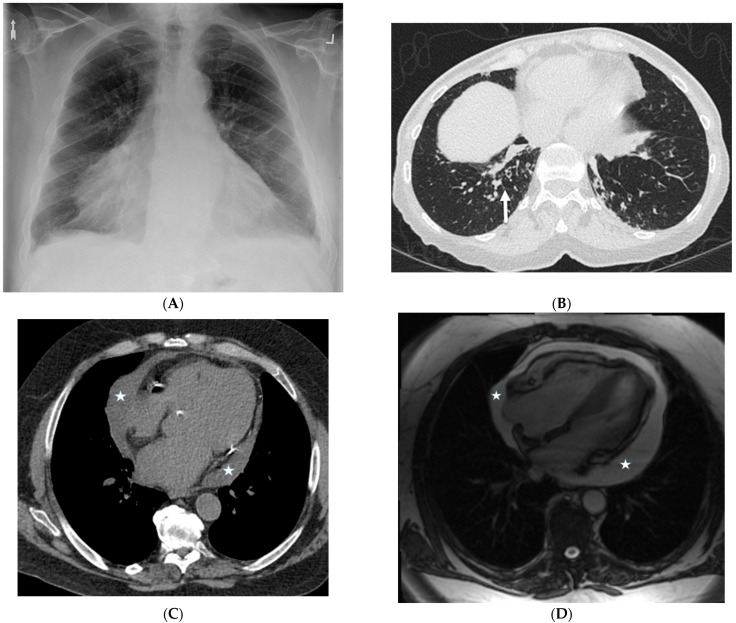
A 75-year-old man with a history of yellow nail syndrome presenting with chronic bronchitis and bronchiectasis. A frontal chest radiograph (A) shows an enlarged cardiac silhouette. An axial chest CT image (B) demonstrates cylindrical bronchiectasis with bronchial wall thickening and foci of peripheral mucoid impaction in lung bases and subtle interlobular septal thickening (solid white arrow) in the left lower lobe. An axial chest CT image (C) and axial bright-blood MR image (D) confirm moderate pericardial effusion (stars).
